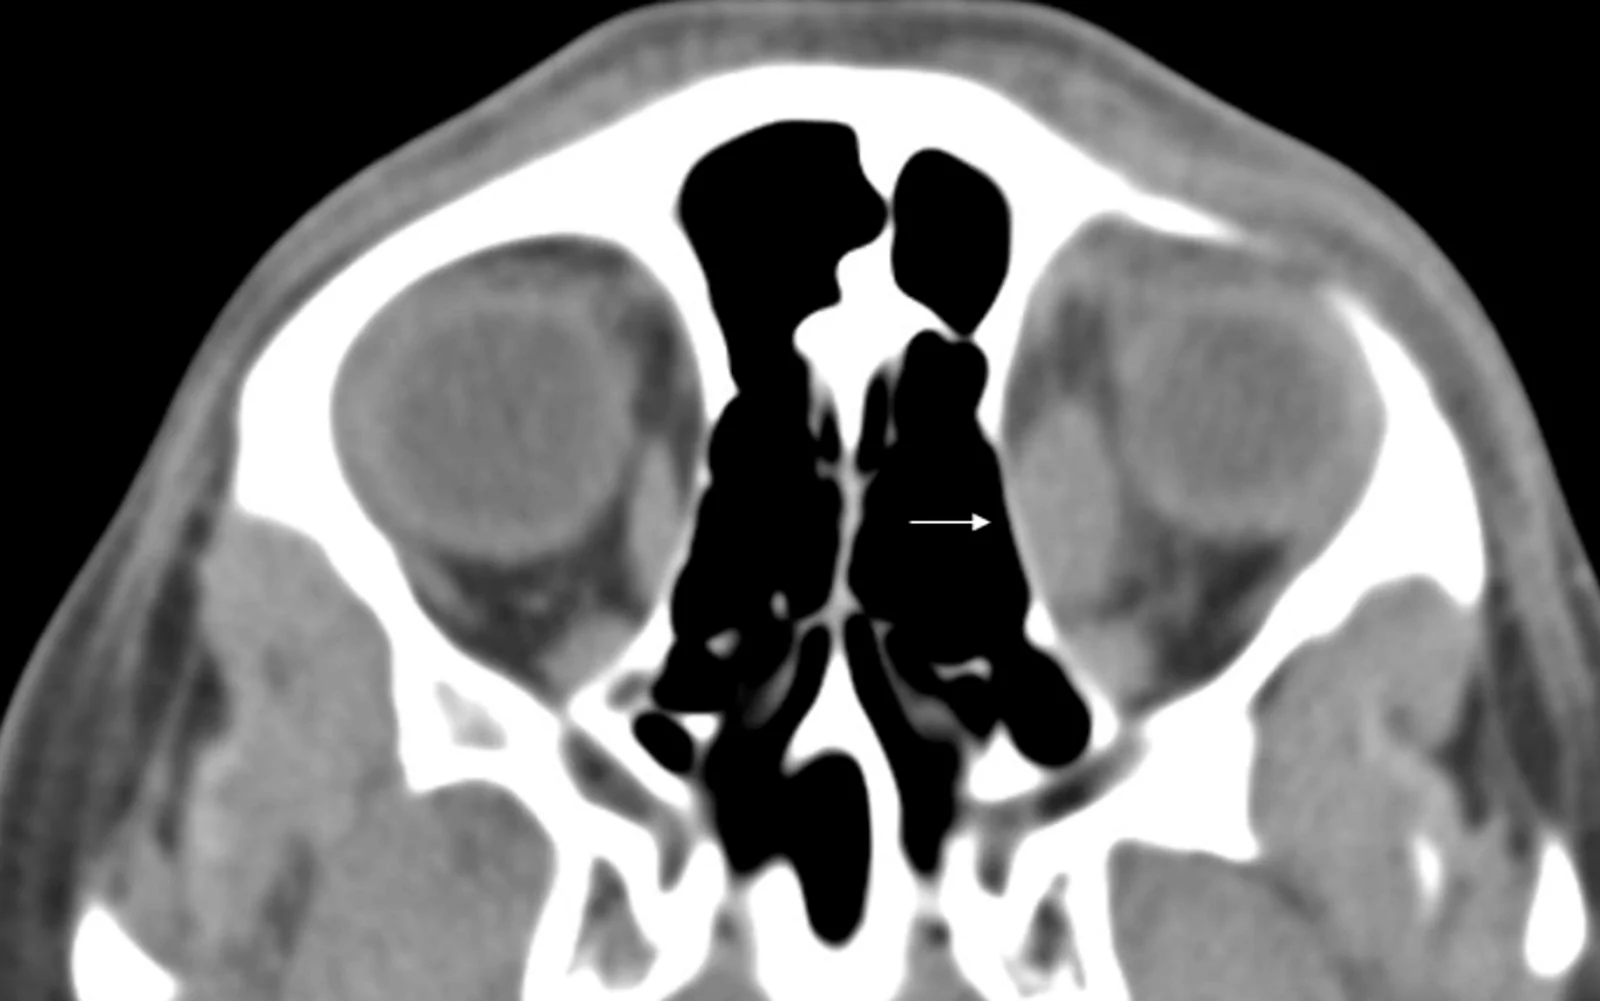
Coronal orbital CT demonstrating fusiform enlargement of the left medial rectus muscle (arrow) with associated mild inflammatory changes in the adjacent medial orbital soft tissues. No evidence of orbital abscess, orbital mass, bony destruction, or paranasal sinus disease is observed.
